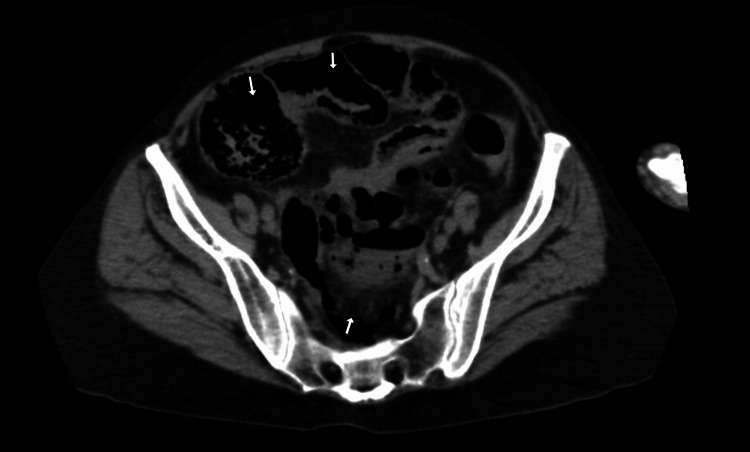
CT Scan Abdomen and Pelvis without contrast - Cross-sectional view- Air in the peritoneum due to intestinal perforation
